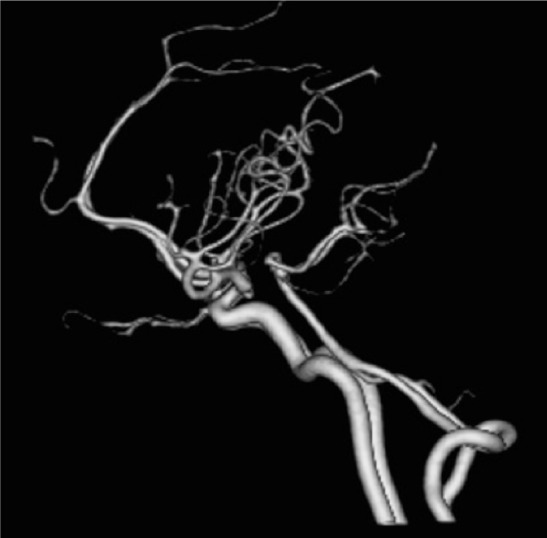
Lateral view CTA 3D reconstruction done at admission.
